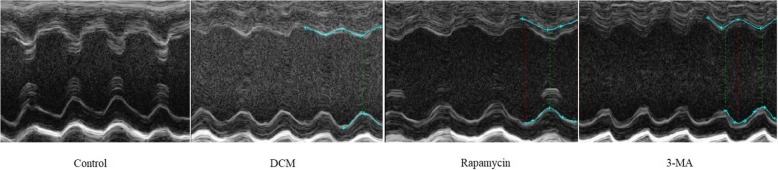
M-mode ultrasound images at the level of papillary muscles in the long-axis view. The M-mode echocardiography evaluation included the following four experimental groups as follows: control group, DCM group, rapamycin group, and 3-MA group. The red lines and blue lines indicated the LVEDD and LVESD respectively. Each group, *n* = 8
